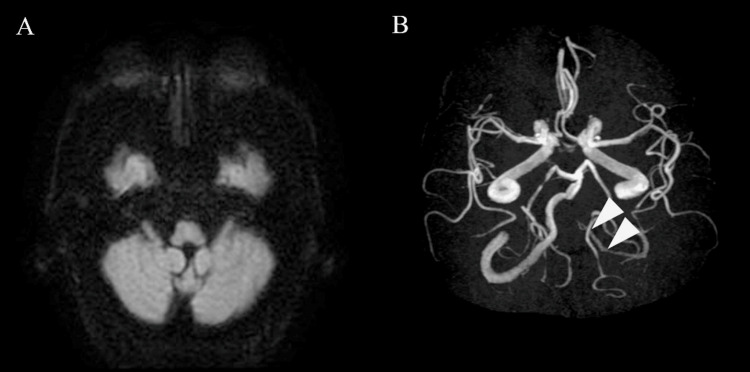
Head MRI findings on the day of admission. A: New high signals were not noticeable on DWI. B: No left vertebral artery delineation on MRA. MRI: magnetic resonance imaging; DWI: diffusion-weighted imaging; MRA: magnetic resonance angiography
